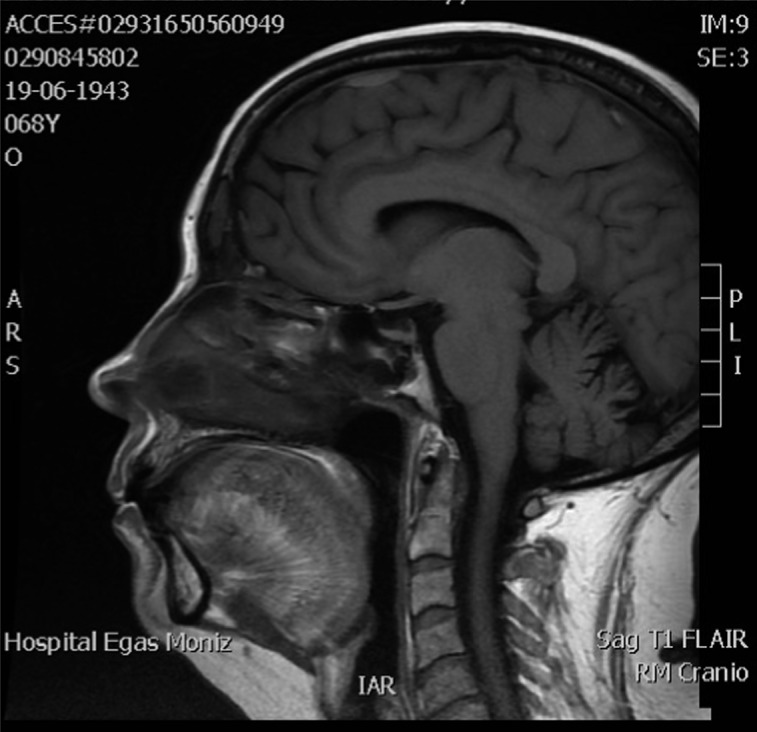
Post-operative cranial MRI.
